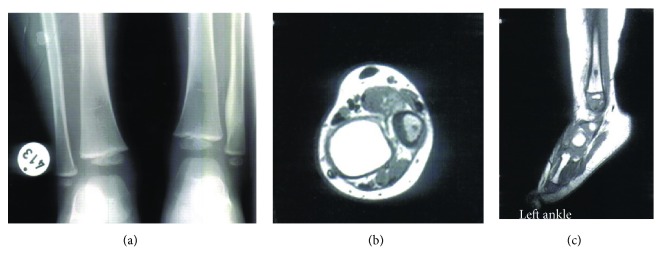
(a) X-ray with thickening of the distal part of the lateral malleolus. (b, c) MRI with the nidus of the OO on the longitudinal and transverse planes.
